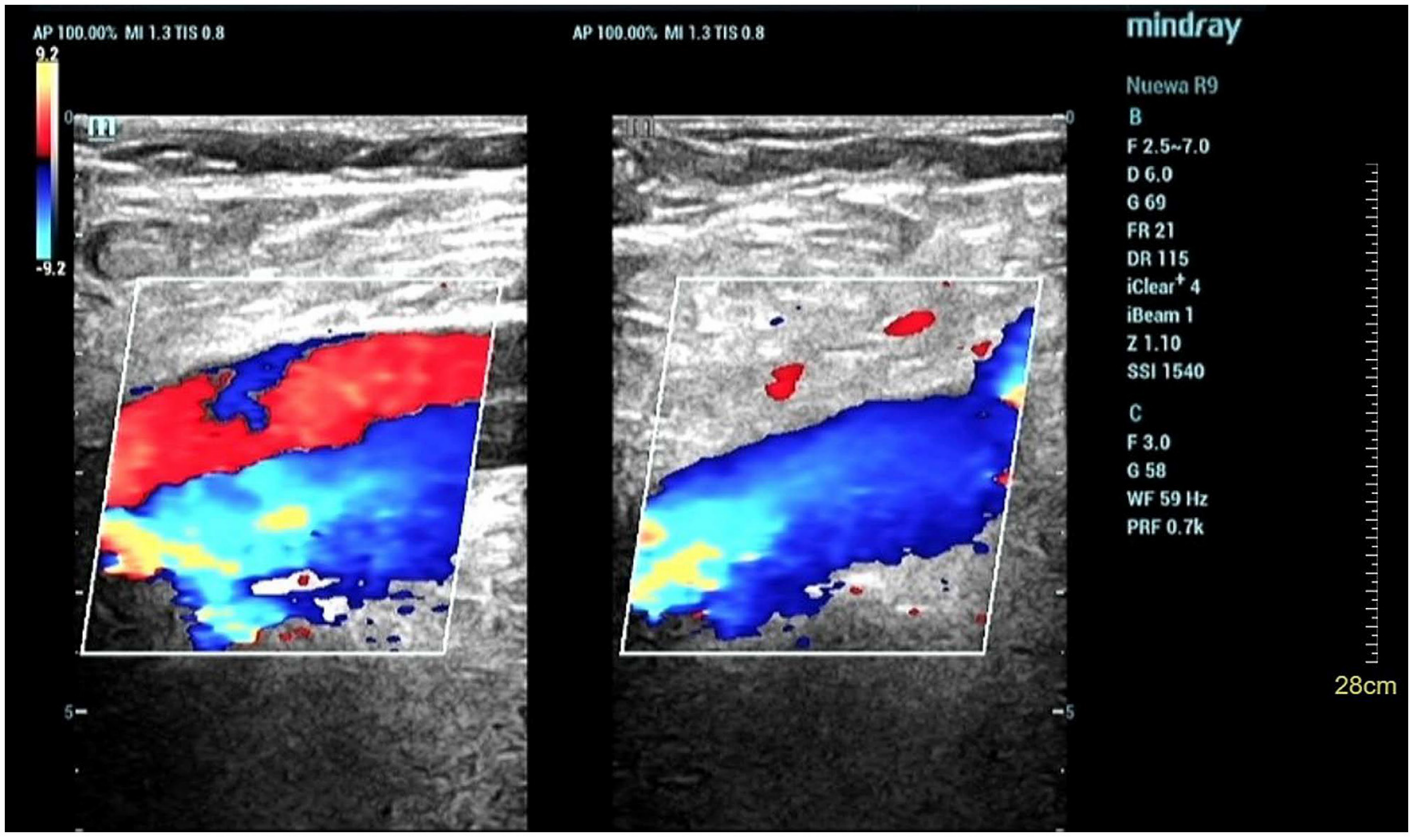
Ultrasonic testing showed venous blood flow in the lower extremities is smooth.
